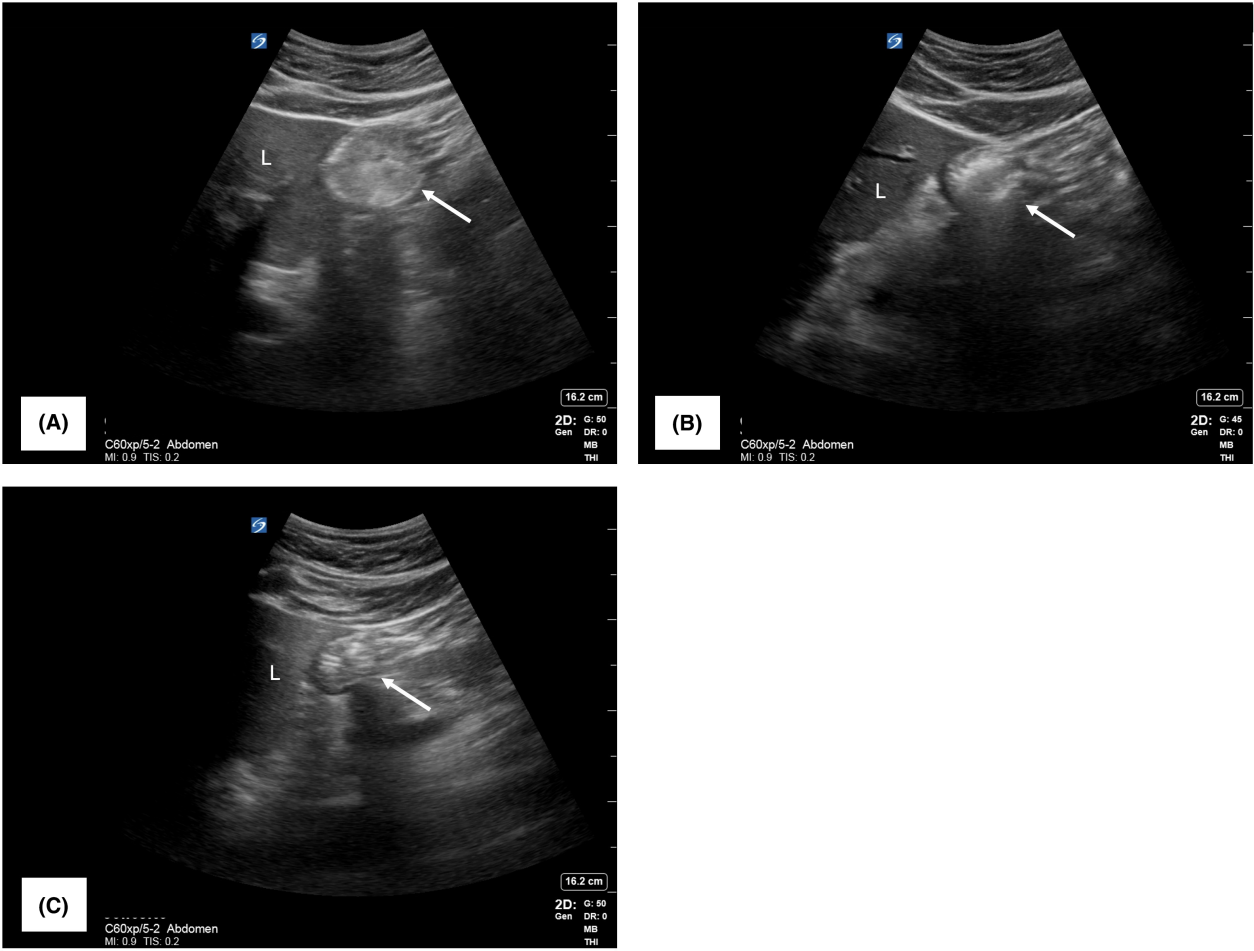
Effect of intravenous erythromycin on gastric emptying in a fasted patient taking GLP‐1 RA assessed using gastric point‐of‐care ultrasound. All images obtained in right lateral decubitus position using a Sonosite™ PX ultrasound machine with a 5‐1 MHz curvilinear transducer (Sonosite, Bothell, Washington, US) on ‘abdominal’ setting at identical depth. (A) Distended gastric antrum with solid content (grade 3). (B) Fifteen minutes after administration of intravenous erythromycin 300 mg, showing a less distended antrum. (C) One hundred minutes after erythromycin administration, gastric antrum is empty (grade 0). GLP‐1 RA, glucagon‐like peptide‐1 receptor agonist; L, liver.
